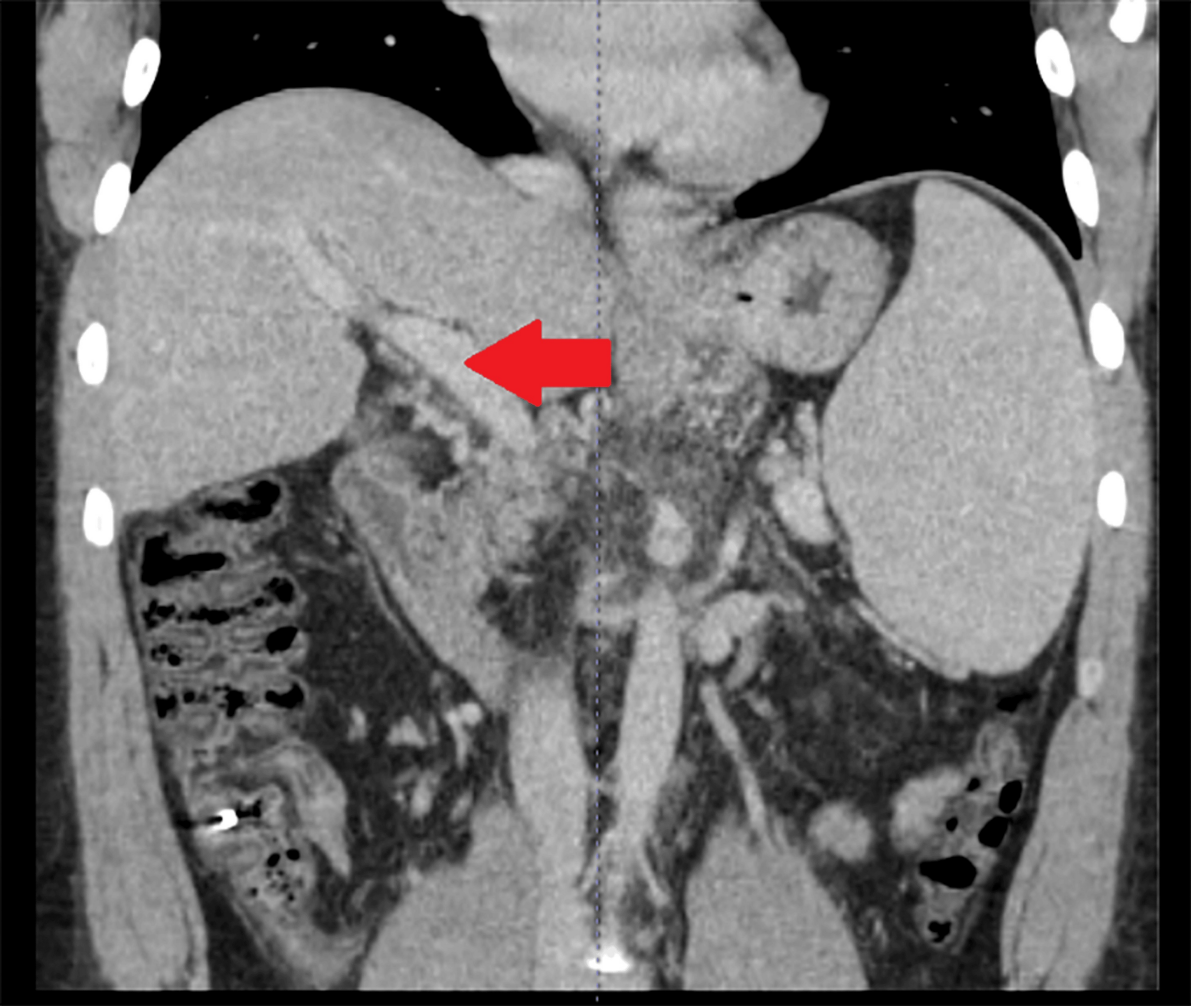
Computed tomography scan abdomen and pelvis 8 weeks after the TIPS procedure Red arrow shows improved clot burden after TIPS and anticoagulation. TIPS: Transjugular Intrahepatic Portosystemic Shunt
